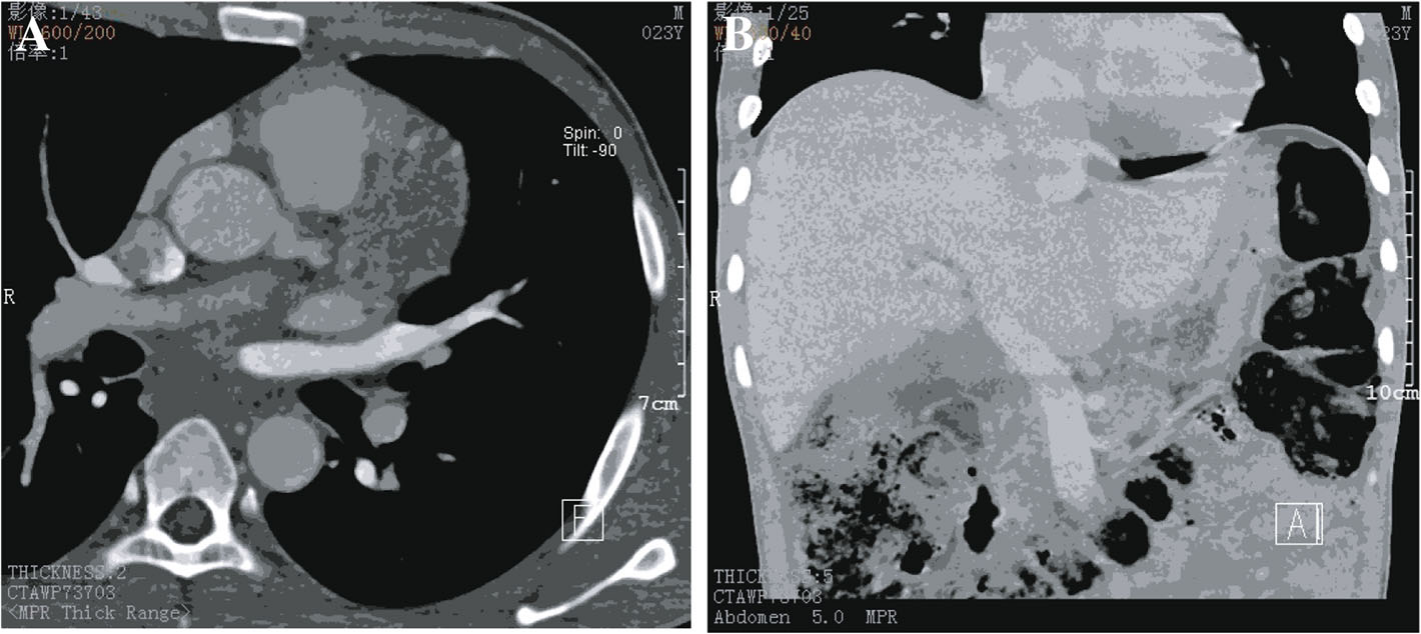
a. Contrast-enhanced CT revealed tumor thrombus extended into right atrium on horizontal scan of heart. b. Contrast-enhanced CT revealed the distance of tumor thrombus was 13.5 cm on longitudinal scan
